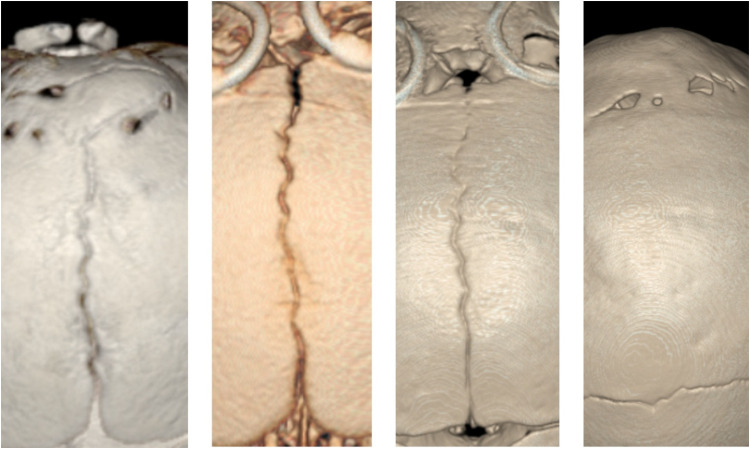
3D CT Images of Sagittal Suture Across Treatment. From Left to Right: Pre Op, Postop Day 2, Pre Spring Removal, Post Spring Removal.
